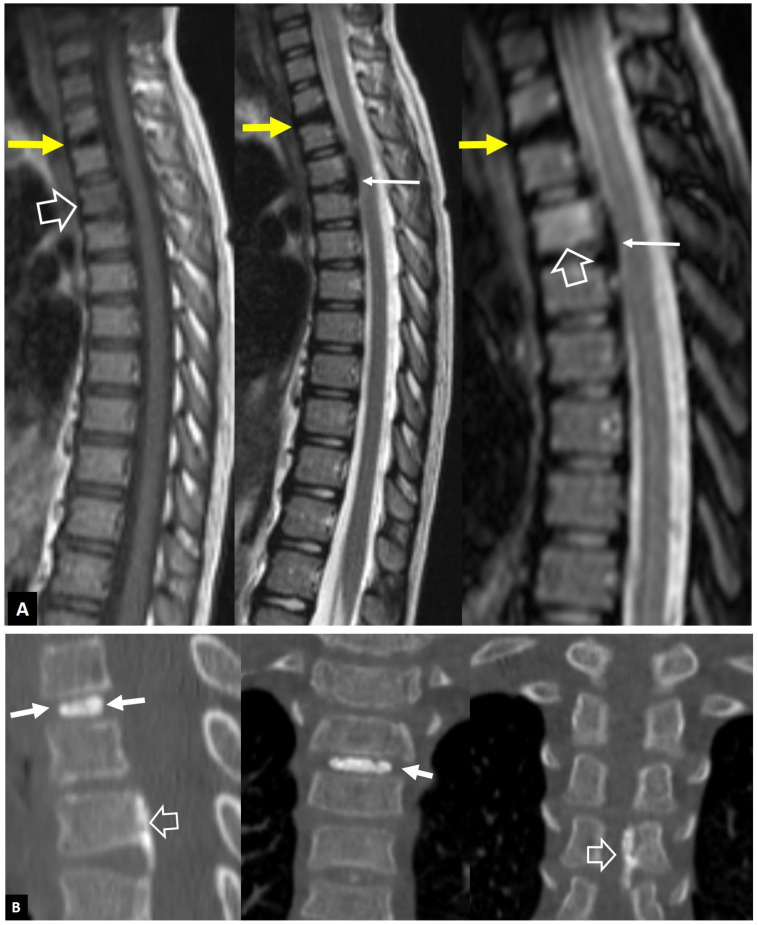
The calcification of the intervertebral disc and the ossification of the posterior longitudinal ligament in a 7-year-old girl presenting with central and left-sided neck pain for 2 weeks and with moderate alleviation with analgesics. The biochemical workup showed an ESR of 65 mm/h (normal up to 20), a CRP of 12 mg/L (normal < 5), and a normal WBC. (A) Sagittal T1-w (left), T2-w (center), and STIR (right) MR images showing the low signal intensity within the disc T2-T3 (arrows), the low signal and thickening of the posterior longitudinal ligament (thin arrows), and the bone marrow edema within the T4 vertebral body (open arrows). (B) CT reconstructions in the sagittal (left) and coronal (center, right) showing the intervertebral T2-T3 disc calcification (arrows) and the ossification of the posterior longitudinal ligament (open arrows) at the T4 vertebra and the T4–T5 intervertebral space.
